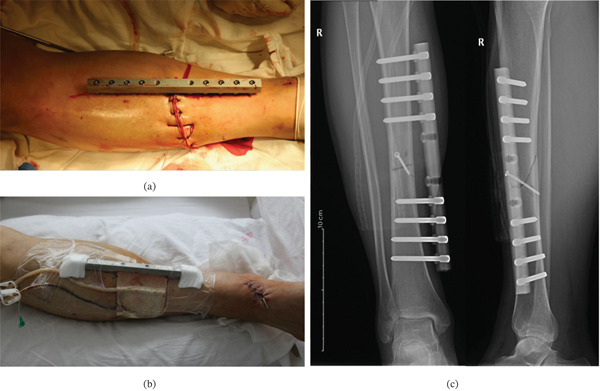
(a) The tibial fracture was stabilized with the modified external fixation and the wound was sutured. (b) The frame and wound were covered by VSD. (c) Anteroposterior radiograph obtained on postoperative Day 1 showing fracture stabilization with the modified external fixation device and lag screws.
